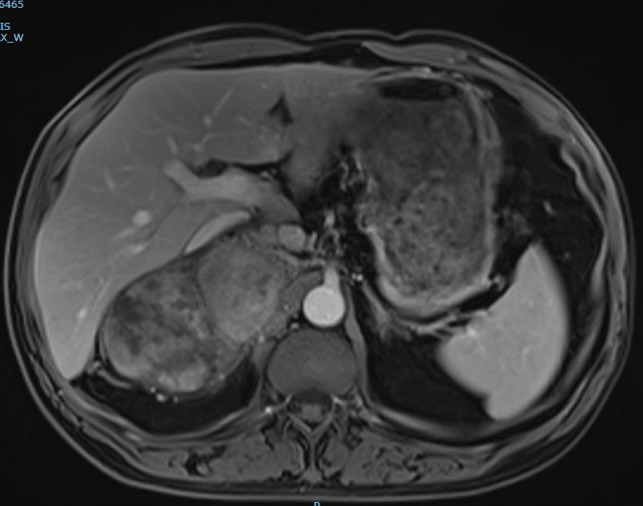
T1-weighted MRI image with gadolinium injection.
